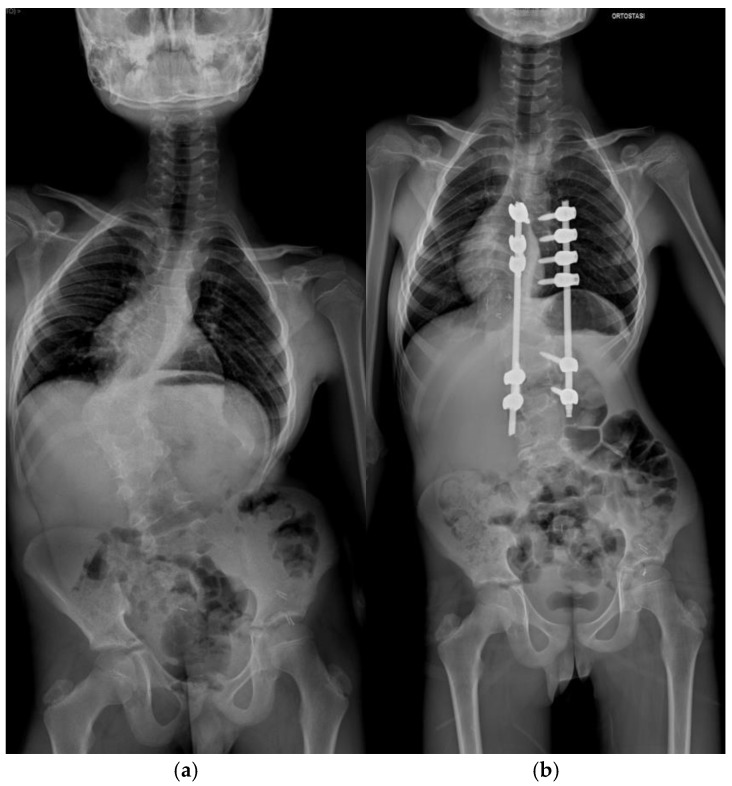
Antero-posterior radiograph of Patient 2. (a) Preoperative assessment. (b) Postoperative assessment.
